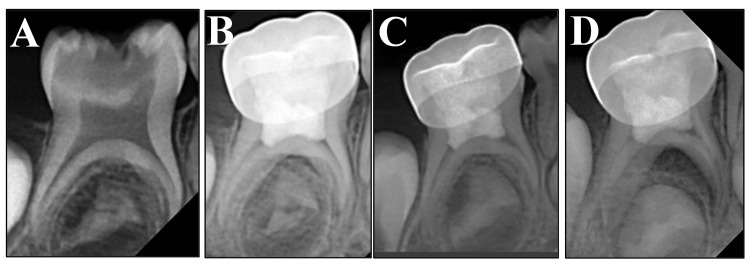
Periapical radiographs of a successful case. (A) Periapical radiograph of a second primary molar showing extensive occlusal caries. (B) Immediate postoperative radiograph after the pulpotomy procedure. (C) and (D) 6- and 12-month follow-up periapical radiographs showing no bone or root resorption. Note the continued normal development of the underlying premolar successor tooth crown.
